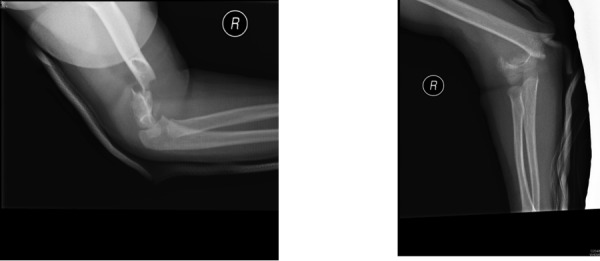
Radiographs of the right elbow demonstrating a supracondylar fracture (Source: K Chan)
